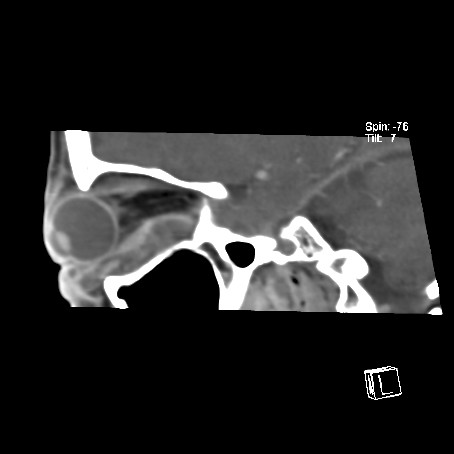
CT scan of the orbits and brain, sagittal view. There appeared to be a more focal peripherally enhancing collection just inferior to the globe and a further enhancing collection adjacent to and within the fossa for the lacrimal sac.
